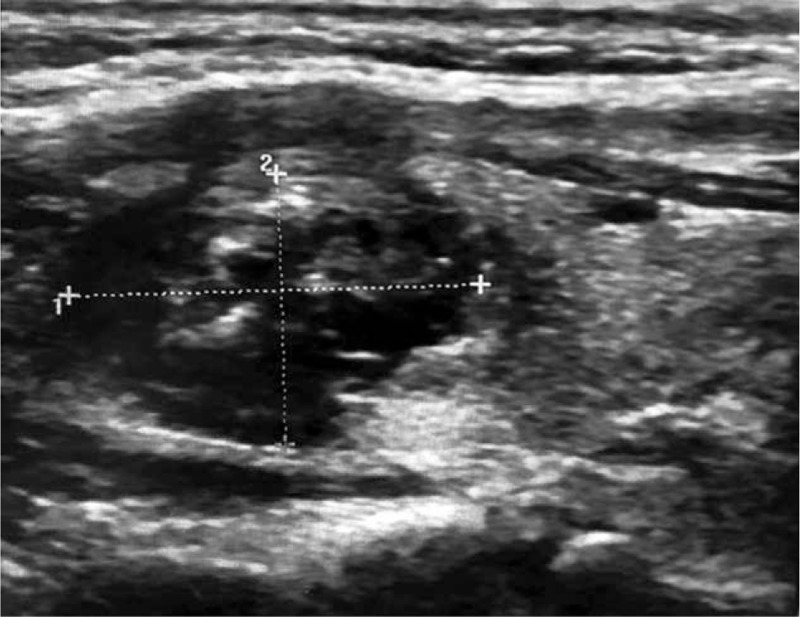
Longitudinal section of the nodule showed by ultrasound after 42 days of MWA. MWA = microwave ablation.
